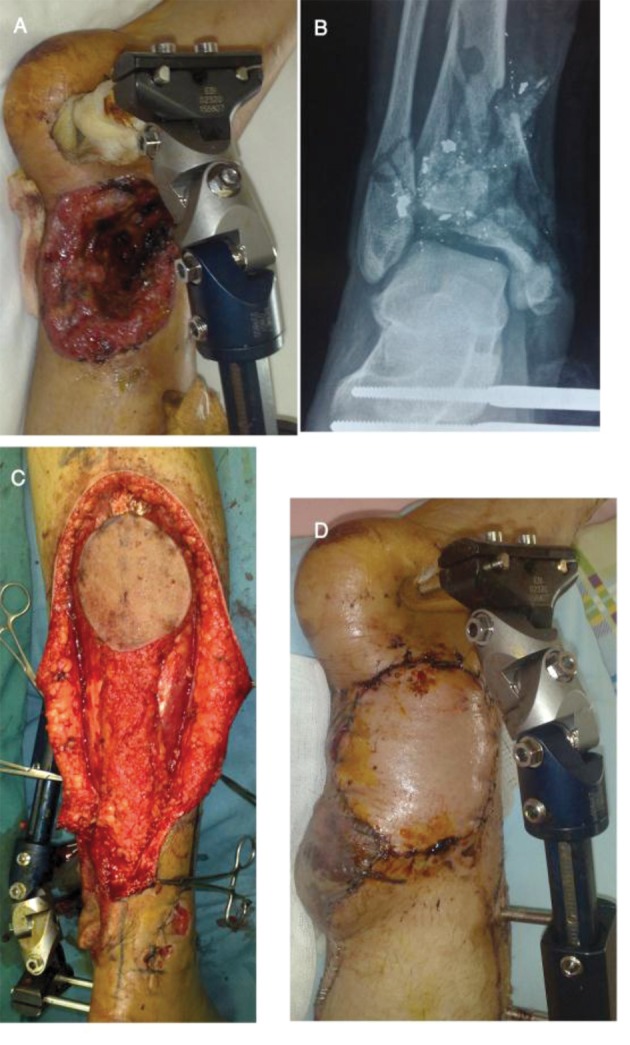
A thirty-five-year-old patient with defects of the lower leg and tibial bone: A-Before reconstruction, B- X-ray view, C-Intra-operative view, D- Follow-up at one month.
